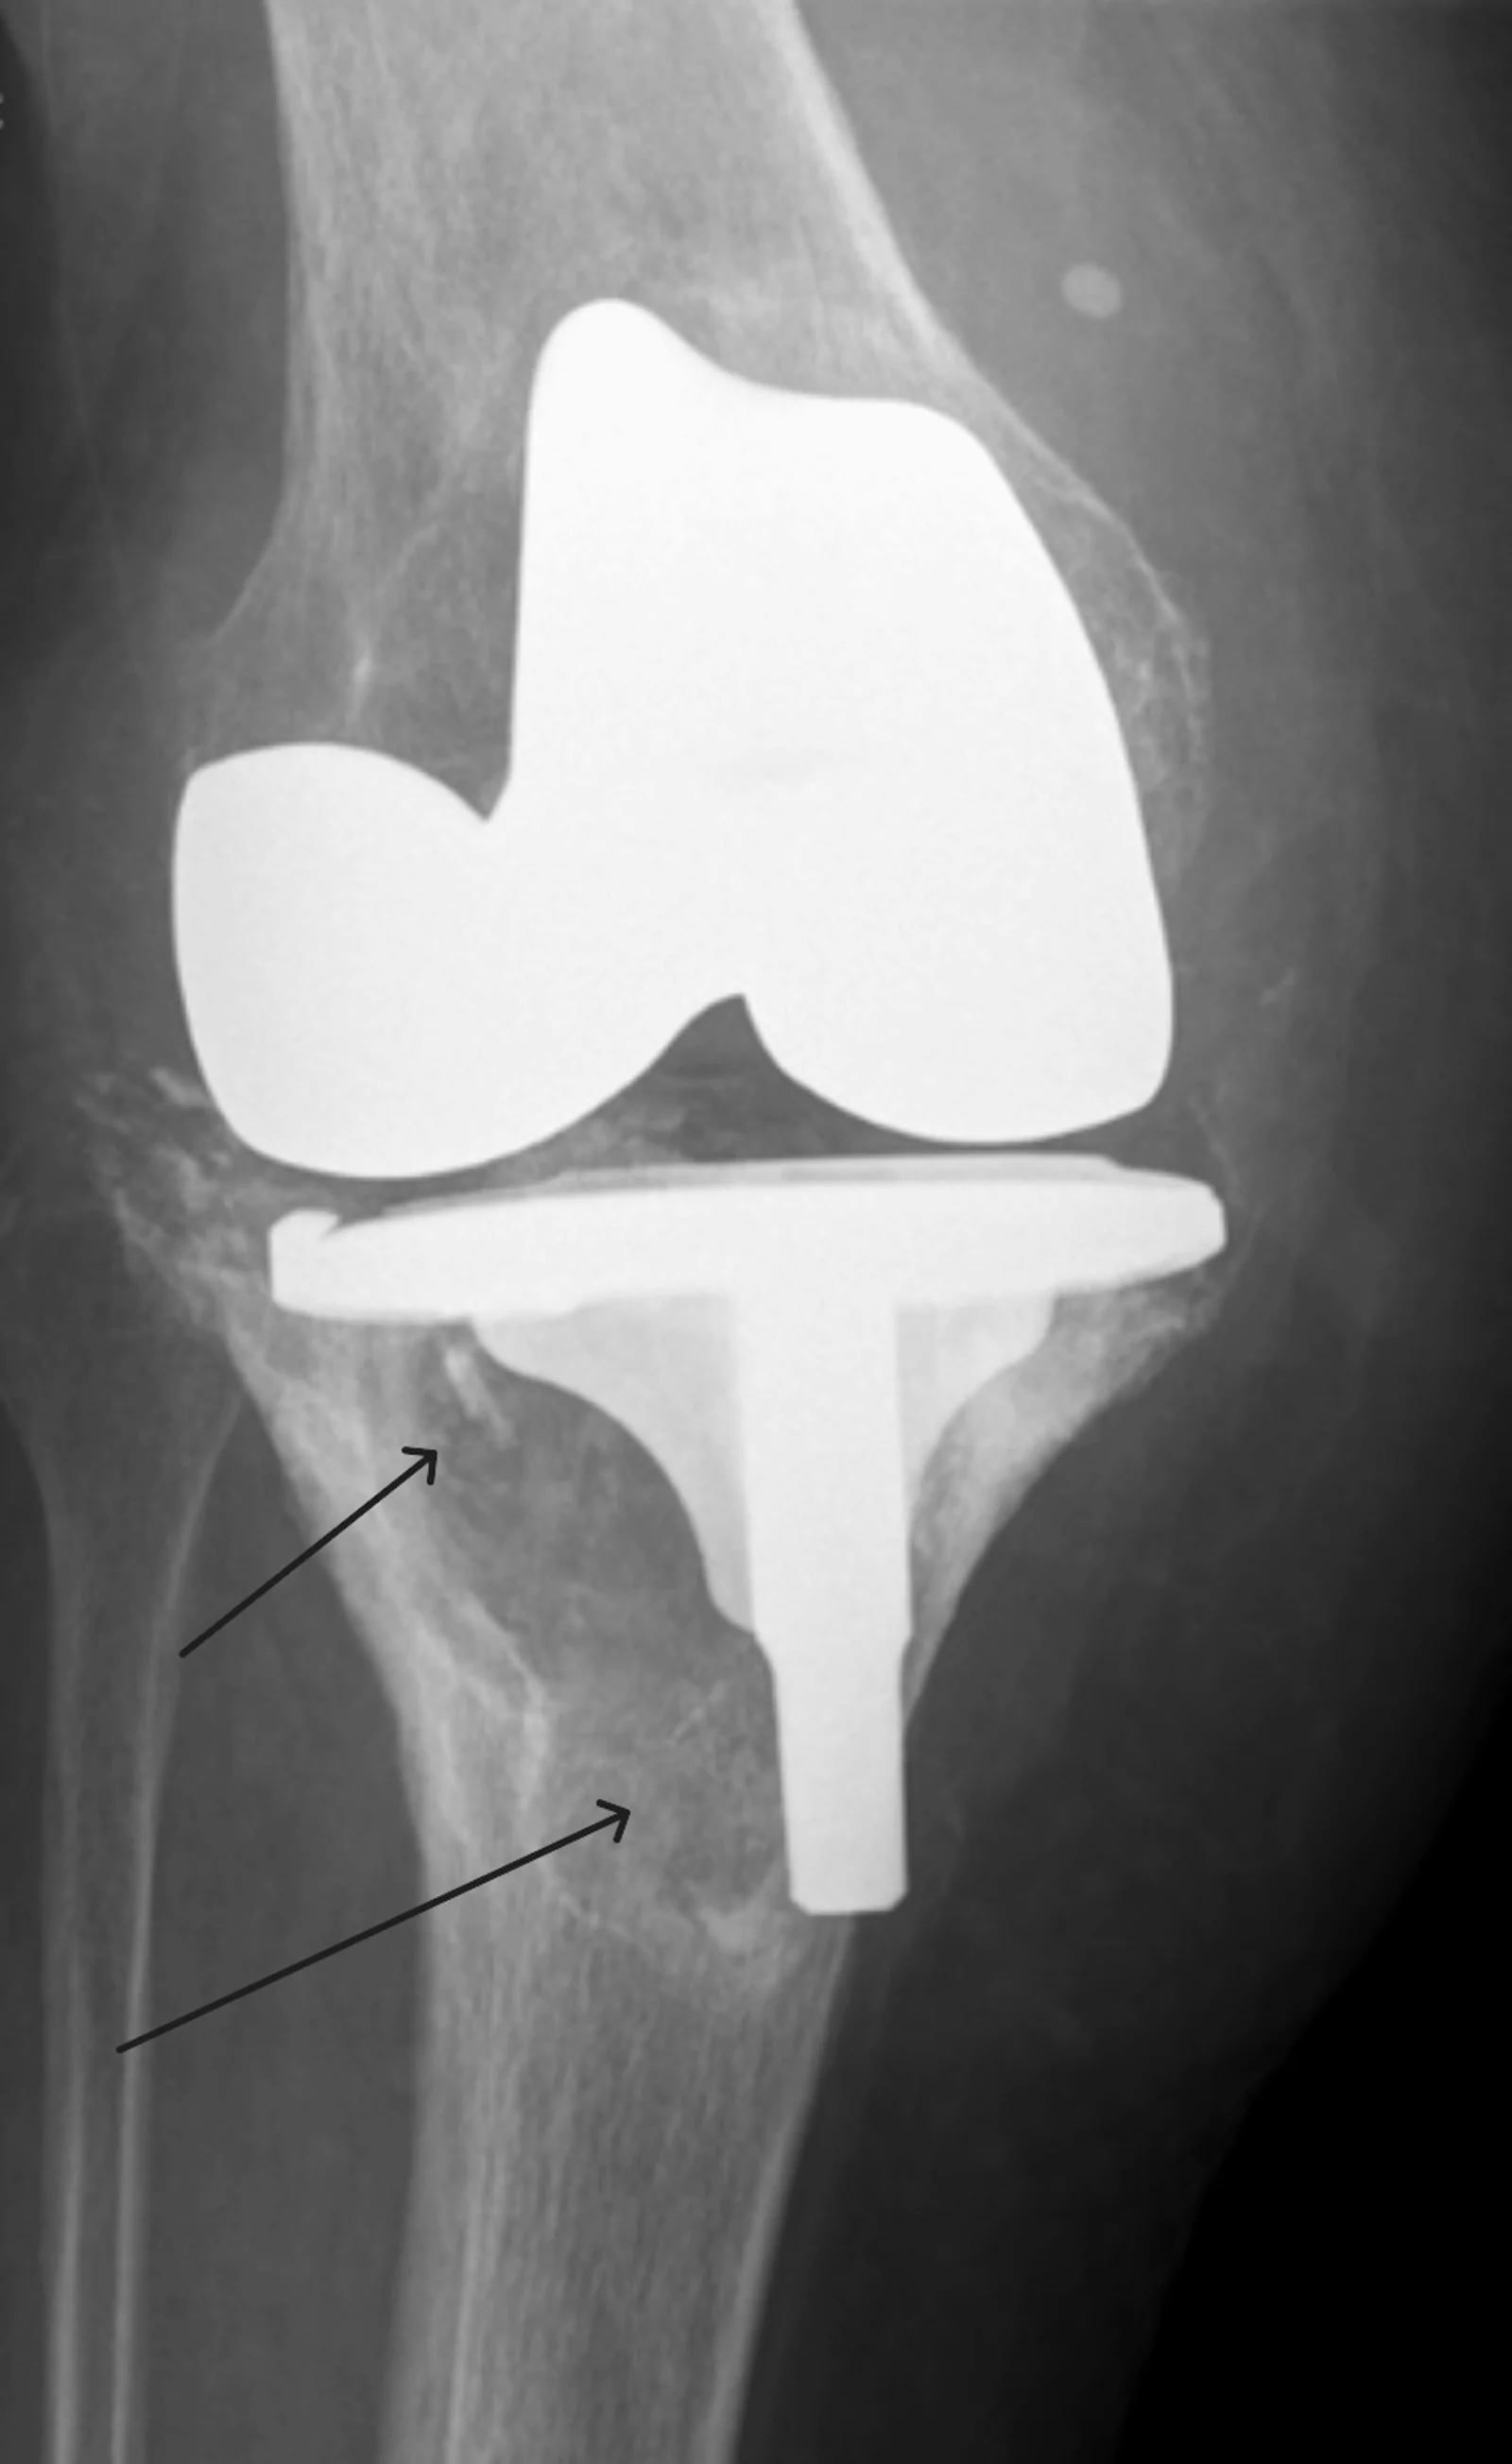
Anteroposterior radiograph of the right knee showing osteolysis of the proximal tibia approximately nine years after primary TKA. TKA, total knee arthroplasty
